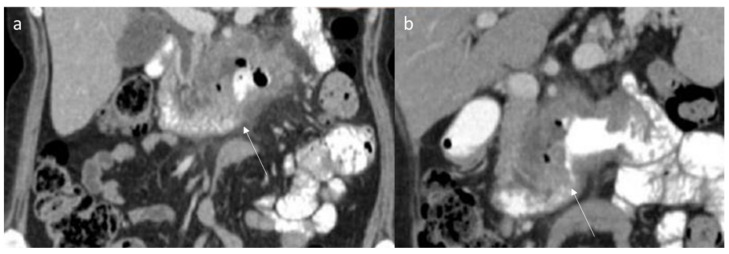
Duodenal carcinoma (arrow) in MPR coronal plane (a,b) of CT assessment during portal phase of contrast study. The duodenum has thickened walls with inhomogeneous contrast enhancement.
